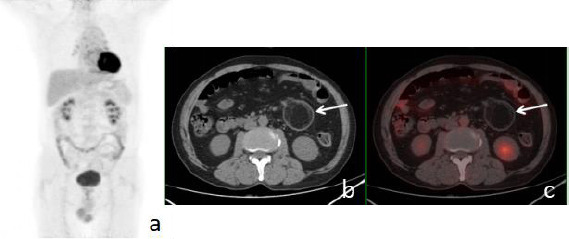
18F-FDG PET/CT of Case 3 showing jejunal lipoma. Abdominal CT before 5 days revealed a small intestinal intussusception, but the PET/CT showed it was revolved. No malignancy was obeserved. (a), PET MIP image; (b), Axial CT; (c), axial PET/CT fusion image.
